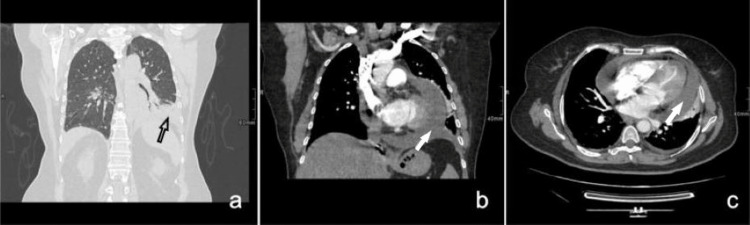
Chest x-ray and computed tomography show consolidation in the posterior and interior parts of the left lower lobe and the lingual of the left upper lobe (black arrow) (a). Large pericardial effusion is present (>3cm) (white arrows) (b,c).
